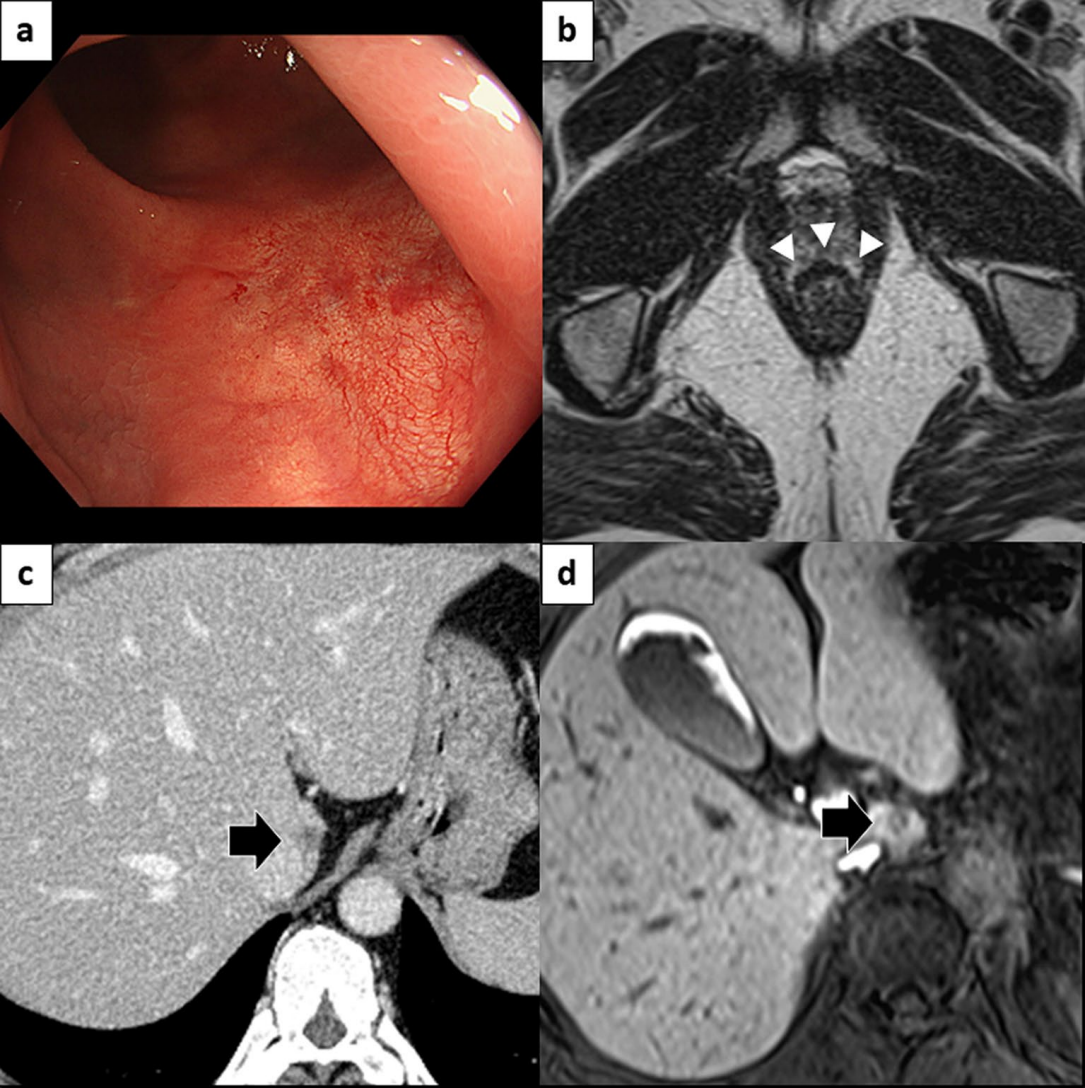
Imaging findings after ICI therapy. a The standard view of the endoscopic image at the lower rectum shows a flat scar without irregularities. b Axial MRI sections of the rectal area, with an arrowhead indicating the scar in the lower rectum with no evidence of residual tumor. c CECT and d EOB-MRI of the liver, with arrows pointing to a significantly shrunken liver metastasis
